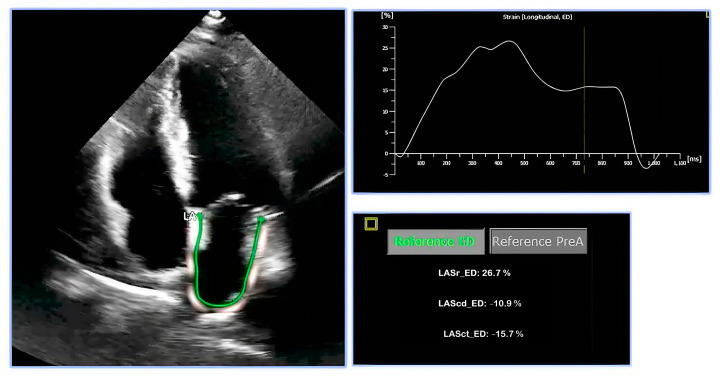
Left atrial strain. The figure shows the different phases of LAS. In the apical four-chamber, begin tracing at the endocardial border of the mitral annulus, follow the LA endocardial border, extending across the pulmonary veins, and/or the orifices of the LA appendage, up to the opposite mitral annulus side. Note, the left atrium is in the far field and, therefore, has reduced image resolution, making it hard to detect speckles. Therefore, we primarily perform contour detection between the blood (black) and the wall (white), followed by calculating length changes. ROI width of 3 mm is recommended, though adjustments should be made to avoid including the pericardium.
